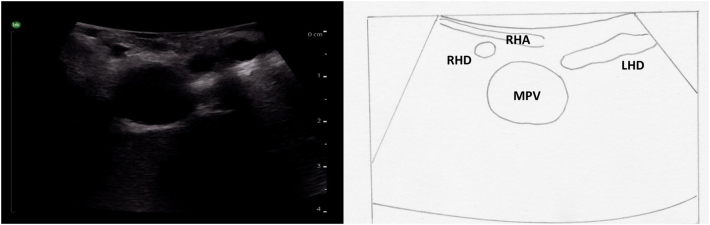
The bifurcation of the left and right hepatic ducts is seen in the superior portion of the hepatoduodenal ligament on intraoperative ultrasound. MPV main portal vein, RHD right hepatic duct, LHD left hepatic duct, RHA right hepatic artery.
